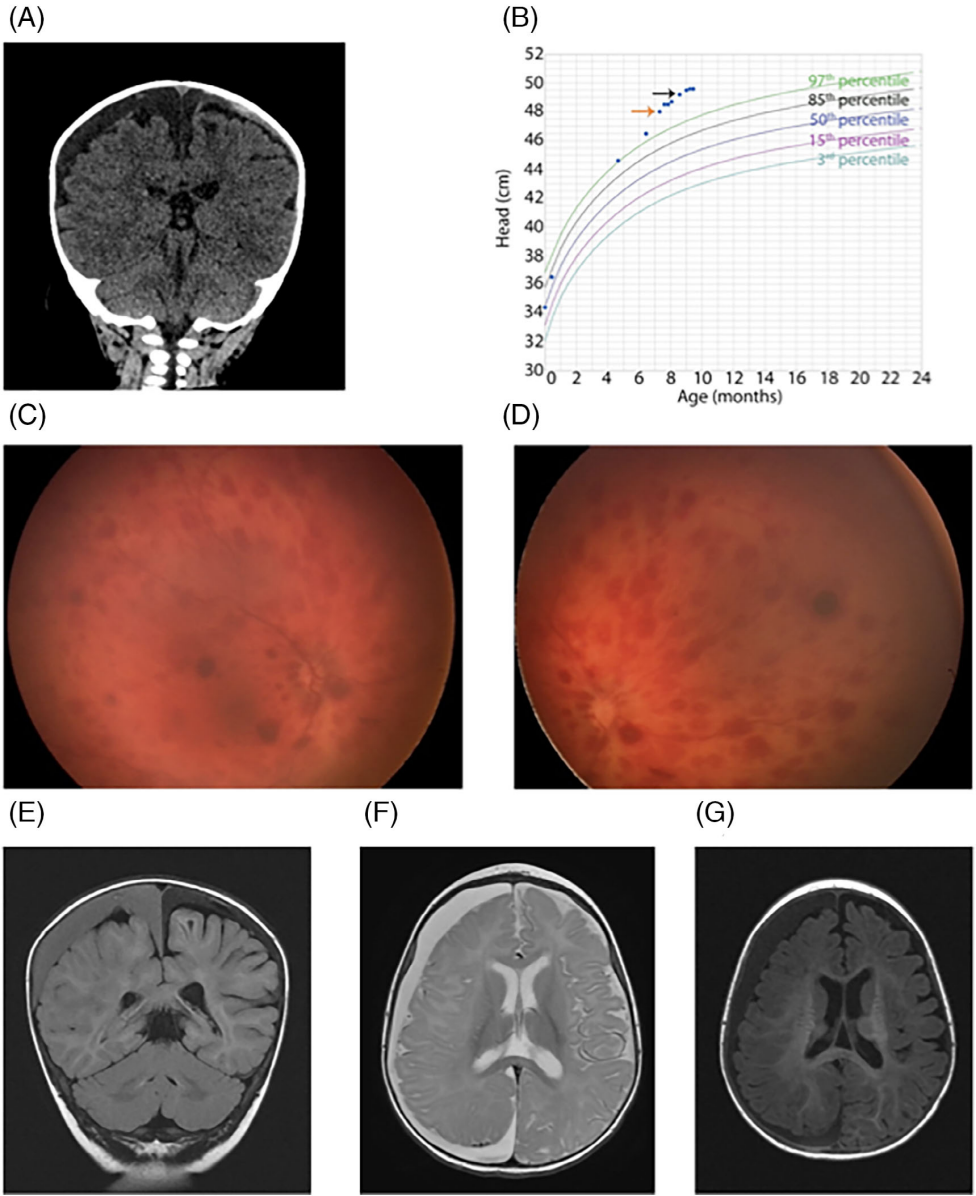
Clinical presentation. A, Computed tomography (CT) head: bilateral subdural hemorrhages of varying hypodensities overly both hemispheres; right brain hypodensity is dark grey, suggesting partial breakdown of chronic blood products; left brain hypodensity has light grey to white overlying dark grey, suggesting an acute on chronic subdural bleed. B, Head circumference measurements during time reported: admission (age 7 months, orange arrow) and second episode (age 9 months, black arrow). C and D, Dilated ophthalmology examination: multilayered intraretinal hemorrhages were observed in the posterior poles of both the right (C) and left (D) eyes. E‐G, MRI brain confirmed bilateral subdural hematomas of different ages across imaging sequences including T2 Flair coronal (E), T2 axial (F), and T1 axial (G). The right convexity subdural hematoma measured 14 mm maximum radial diameter (previously 11 mm on head CT); left subdural hematomas had distinctly different signal characteristics from each other, confirming differing ages. No other brain abnormalities were identified
